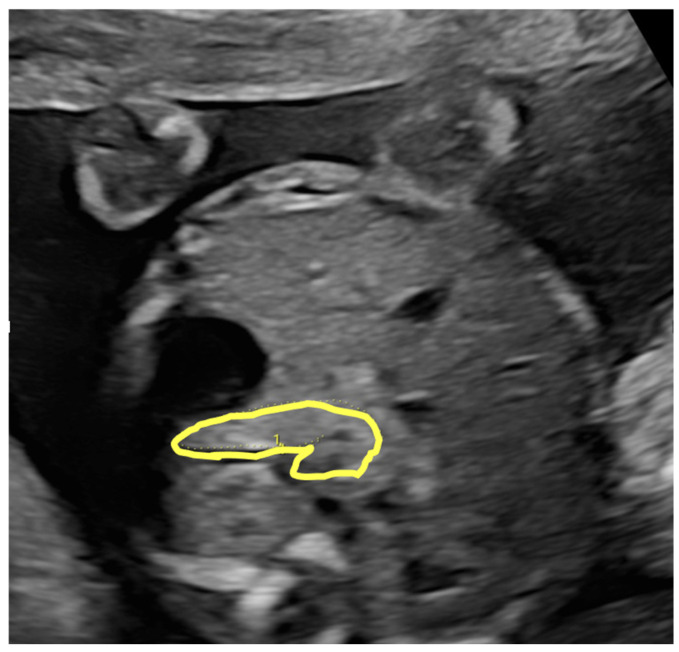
Intrauterine overnutrition: enlarged fetal pancreas preceding gestational diabetes diagnosis. Ultrasound at 22 + 0 weeks demonstrating enlarged fetal pancreas (>90th percentile), with the pancreatic circumference highlighted in yellow, preceding subsequent diagnosis of gestational diabetes mellitus. This fetus with an enlarged pancreas is prone to develop future impaired glucose tolerance.
